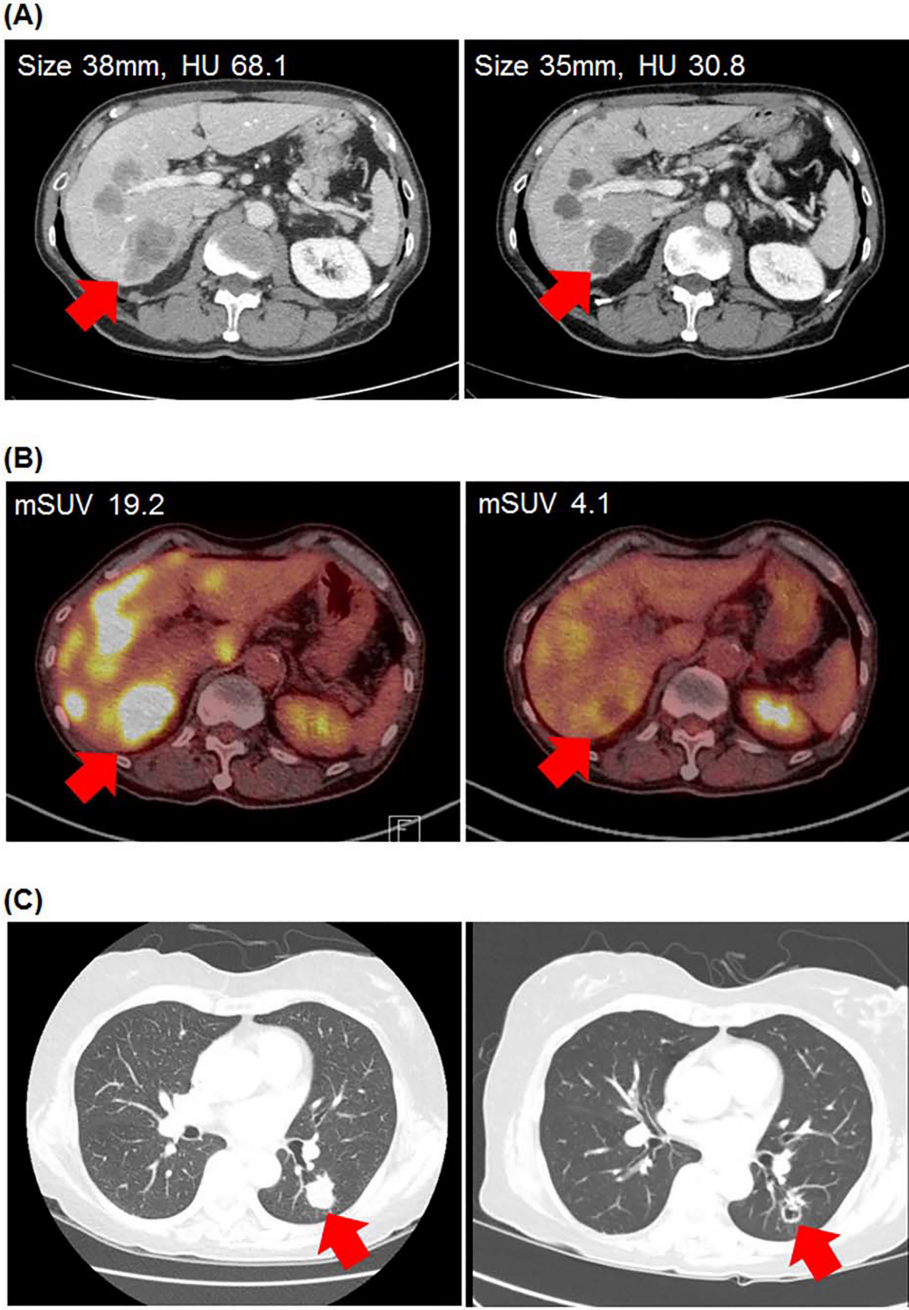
Representative images of anti-angiogenic changes of regorafenib. (A) Tumor size and attenuation in baseline CT (left) and follow-up CT after 2 cycles of regorafenib treatment (right). (B) PET-CT images of the same target lesion before (left) and after 2 cycles (right). (C) Cavitary change of lung metastasis. Images of baseline CT (left) and follow-up CT after 2 cycles (right).
